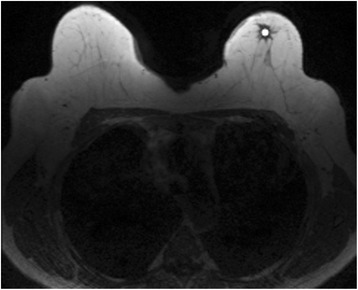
Standard size circular region of interest (ROI) loaded over T1-weighted MR image in MaZda after image normalization. The tumour is grade 3 invasive ductal carcinoma in left breast
